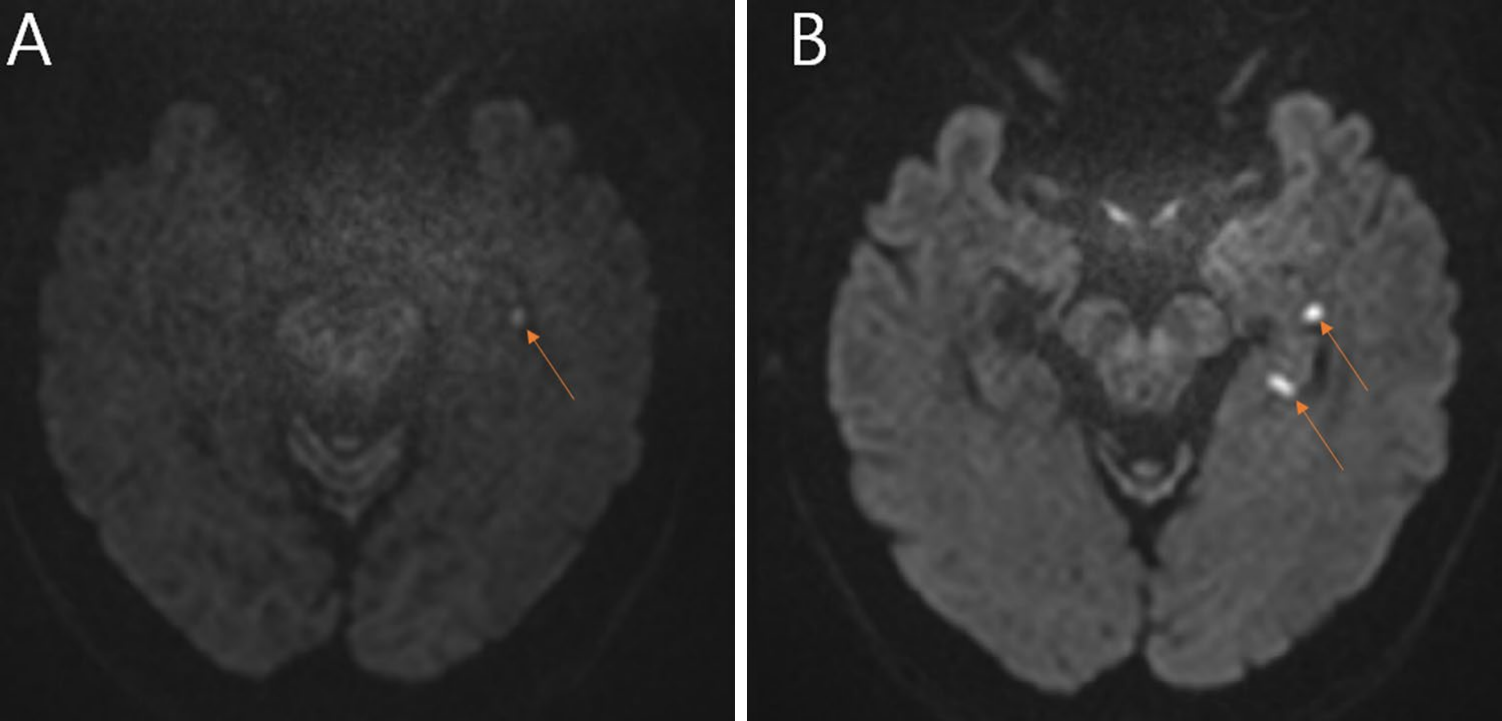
DWI in patient 3. The initial DWI showed a subtle high signal intensity involving the left hippocampal head (A). Follow-up DWI revealed two distinct lesions involving the head and body of left hippocampus (B)
